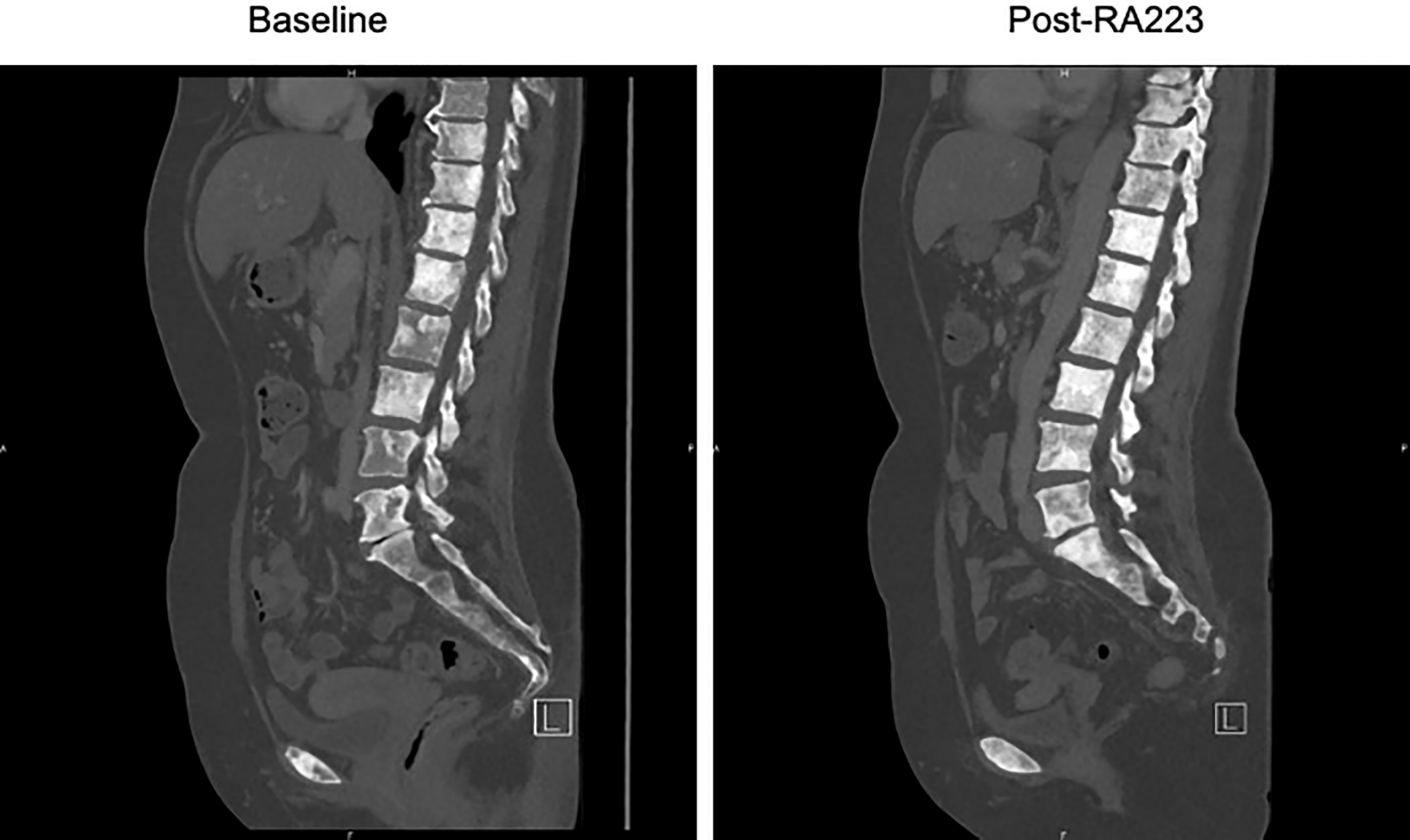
Lumbar spine CT-scan before and after Radium-223 treatment. The CT-scan performed after Radium-223 shows bone formation in lumbar vertebras.
